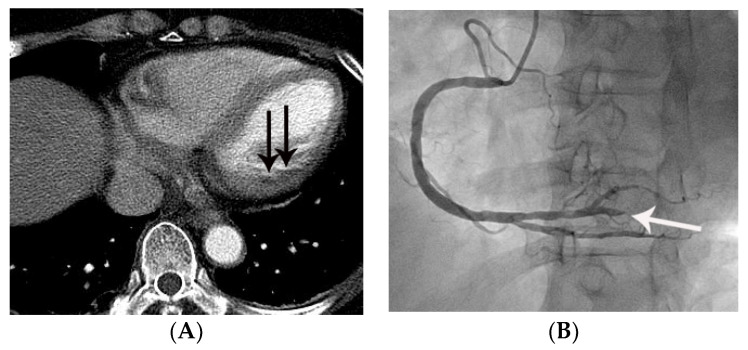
False negative diagnosis of acute myocardial infarction on non-gated chest CT in a 67-year-old female with a small acute myocardial infarction. (A) Equivocal MPD (CT score of 1 for myocardial perfusion defect (MPD)) (arrow) was noted in the basal lateral segment of the left ventricle on an axial image. (B) Subsequent coronary angiography showed total occlusion (arrow) of the posterolateral branch of the right coronary artery, suggesting a culprit lesion. Note that it was difficult to differentiate true MPD from artifacts in this case.
